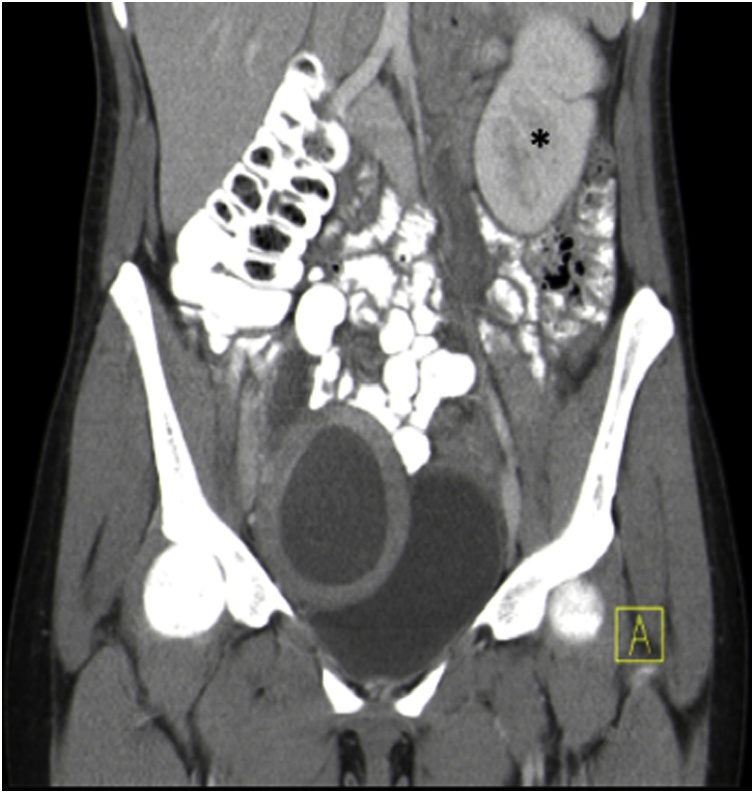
The coronary plane of CT scan with oral contrast: Note only one kidney on patient’s left side (*).
